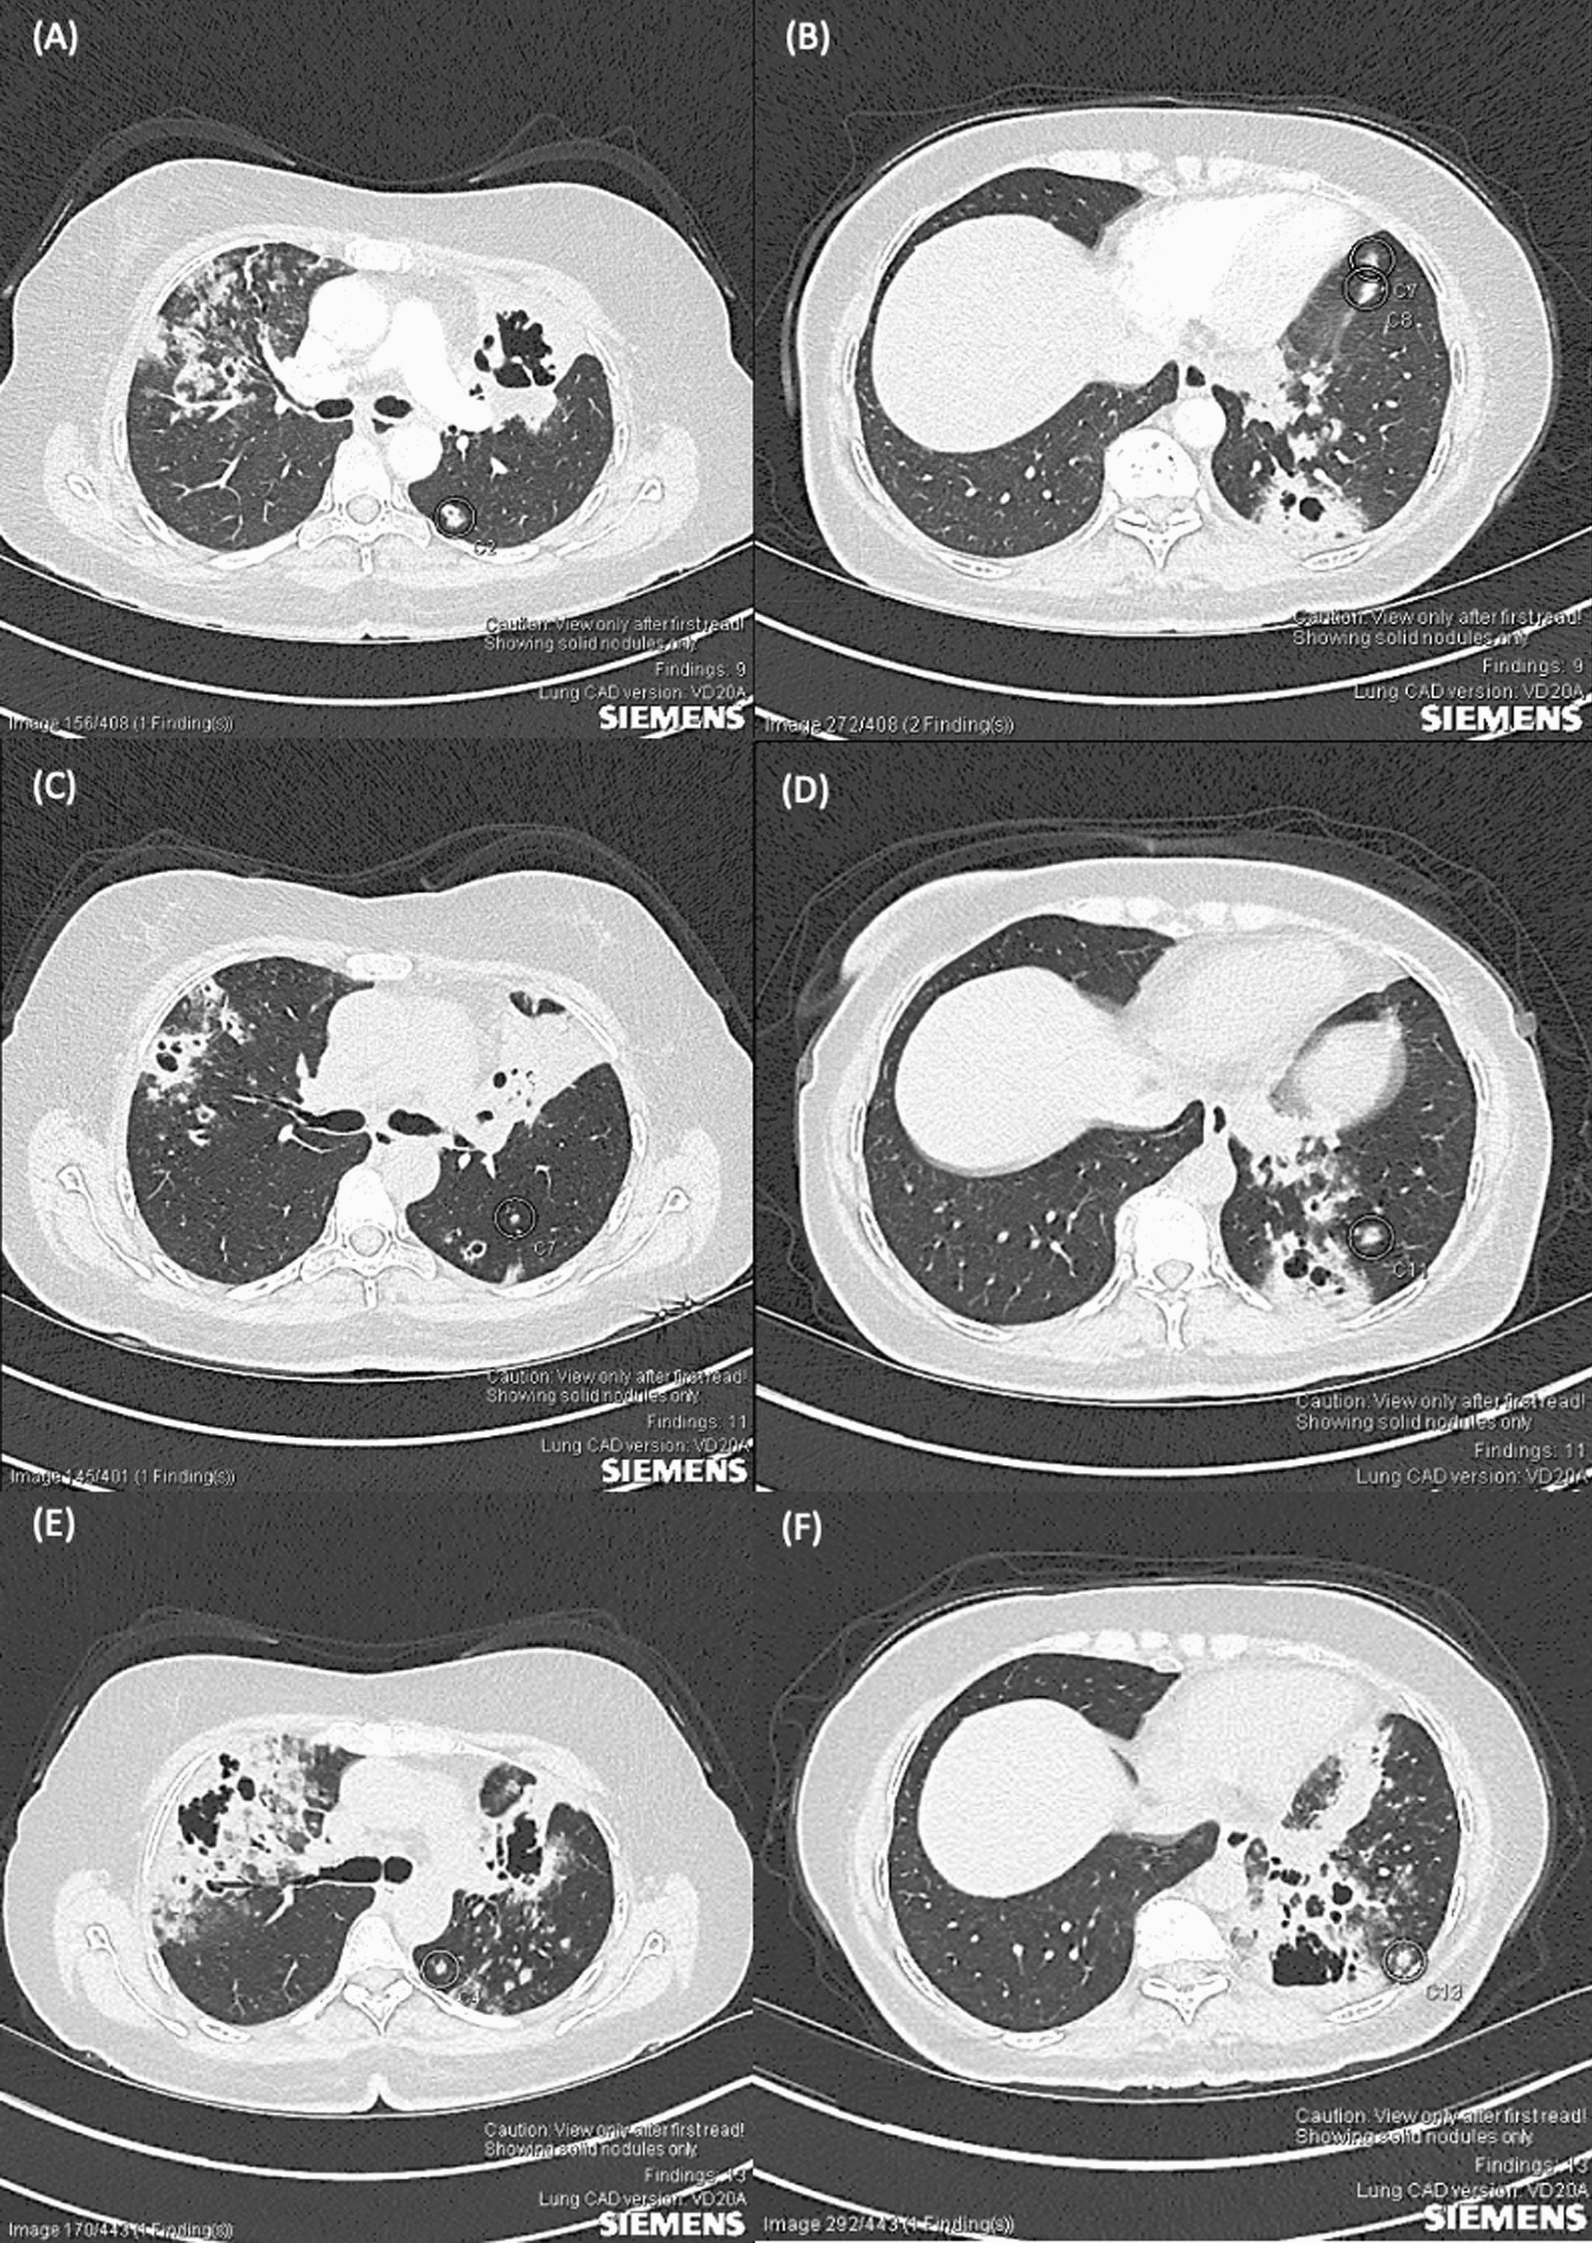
A, B Chest computed tomography pulmonary angiogram scan axial cut on admission showed extensive coarse infiltrates in the upper lobes, with extensive cavitation in the left upper lobe, and multiple small areas of cavitation bilaterally with associated fibrosis in left lower lobe; C, D imaging post-antimicrobials showed reduction in size of the left upper lobe cavitation, resolution of extensive alveolar infiltrates in right upper lobe, and no substantial changes in left lower lobe; E, F repeat imaging showed worsening cavitary lesions and new extensive centrilobular ground-glass nodules in the left upper and lower lobe
